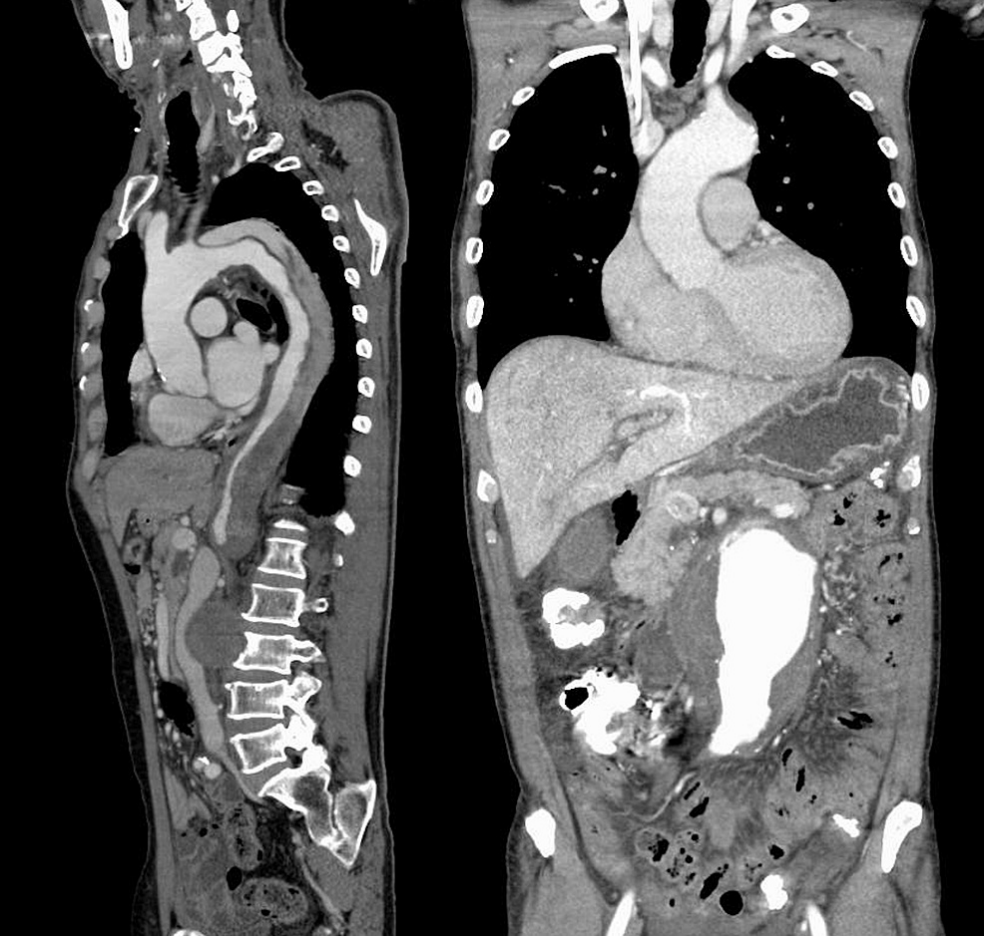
Chest and abdominal CT. Chest and abdominal contrast-enhanced CT show a Stanford type B aortic dissection and abdominal aortic aneurysm. CT: computed tomography
